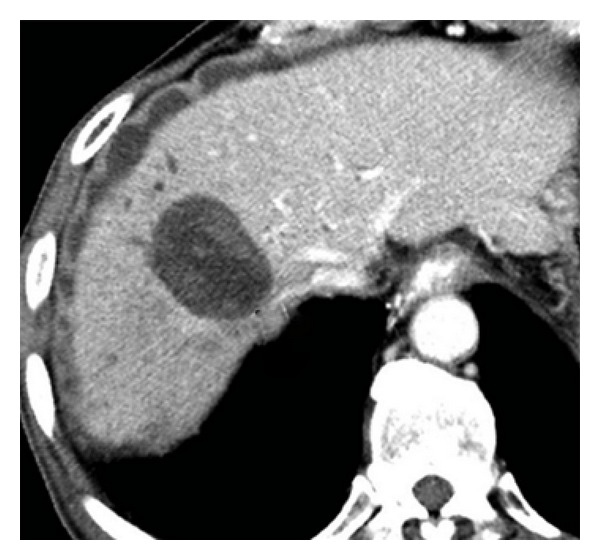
CT scan showing a biloma surrounding the ablated tumor after RF ablation.
